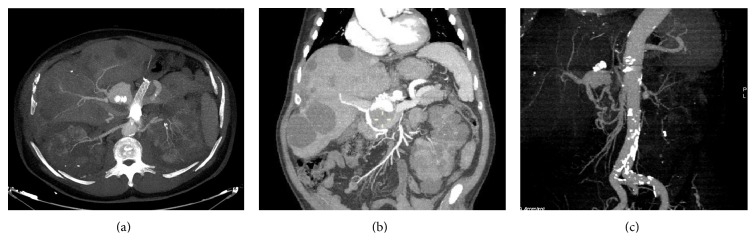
CT scan after 5 years from intervention. (a) MIP reconstruction in the axial plane showing aneurysm relapse in the hepatic artery; patency of the stent in the splenic artery is also evident; MIP (b) and VR (c) reconstructions in coronal and sagittal oblique planes demonstrating the pancreaticoduodenal arcade supplying the aneurysm relapse; the vascular plug, previously positioned, is noted. Renal and hepatic cysts are observable in Figure (b).
